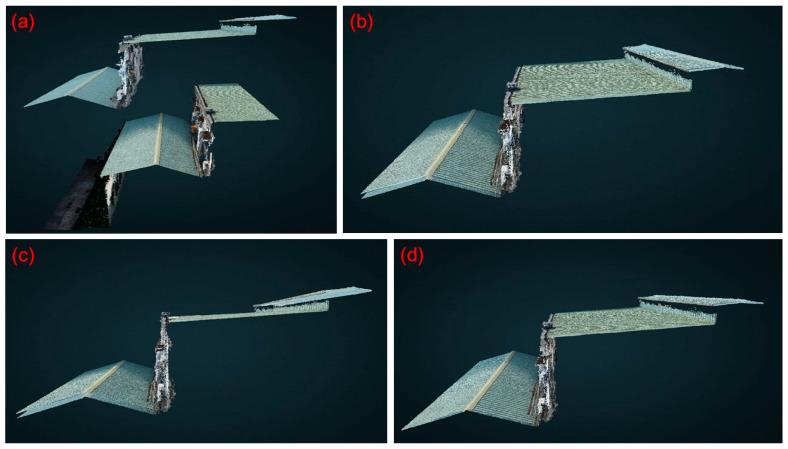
The partial integration results of UAV-based point cloud Models 2-1 and 2-2 in study area 2: (a) without the CT method, (b) with the 4-parameter CT method, (c) with the 6-parameter CT method, and (d) with the 7-parameter CT method. The entire point cloud integration results for (a–d) can be found at the following four links (accessed on 30 August 2024): https://www.pointbox.xyz/clouds/66b37dfb817a428b49556804, https://www.pointbox.xyz/clouds/66b330a2817a42933b5567e6, https://www.pointbox.xyz/clouds/66b33288817a42ba785567e8, https://www.pointbox.xyz/clouds/66b33446817a425bbb5567ea.
